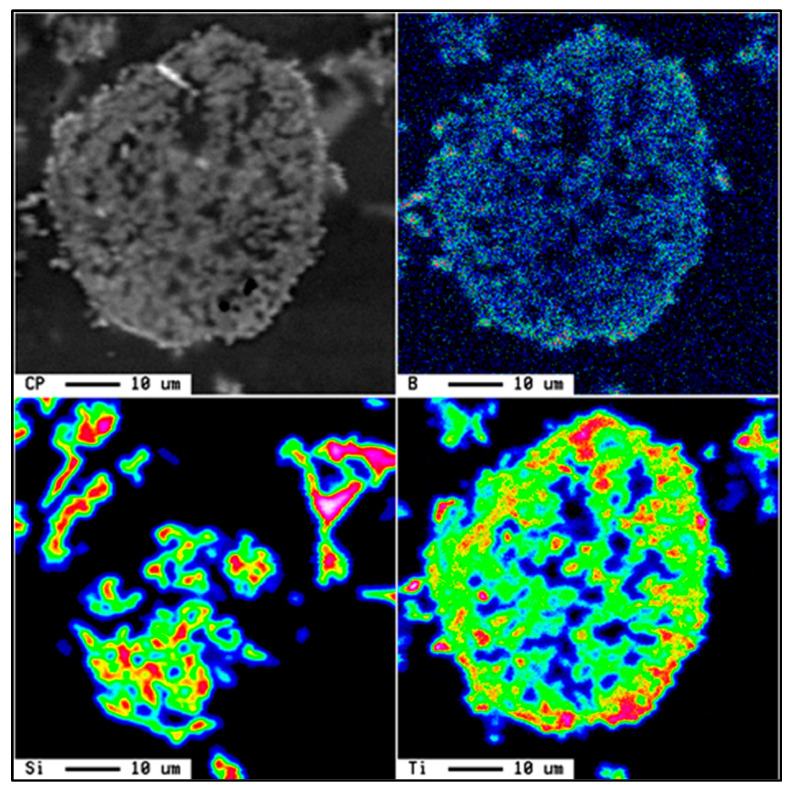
An X-ray map showing the distribution of Ti, Si, and B.
